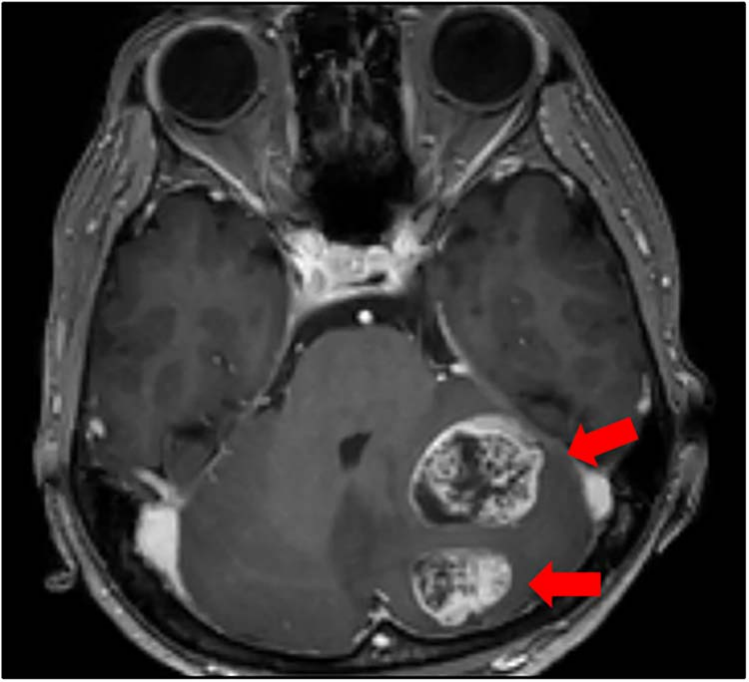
Cranial MRI findings (T1-weighted image). Two ring-enhanced masses with maximum diameters of 3.3 and 2.4 cm were observed in the left cerebellum.
